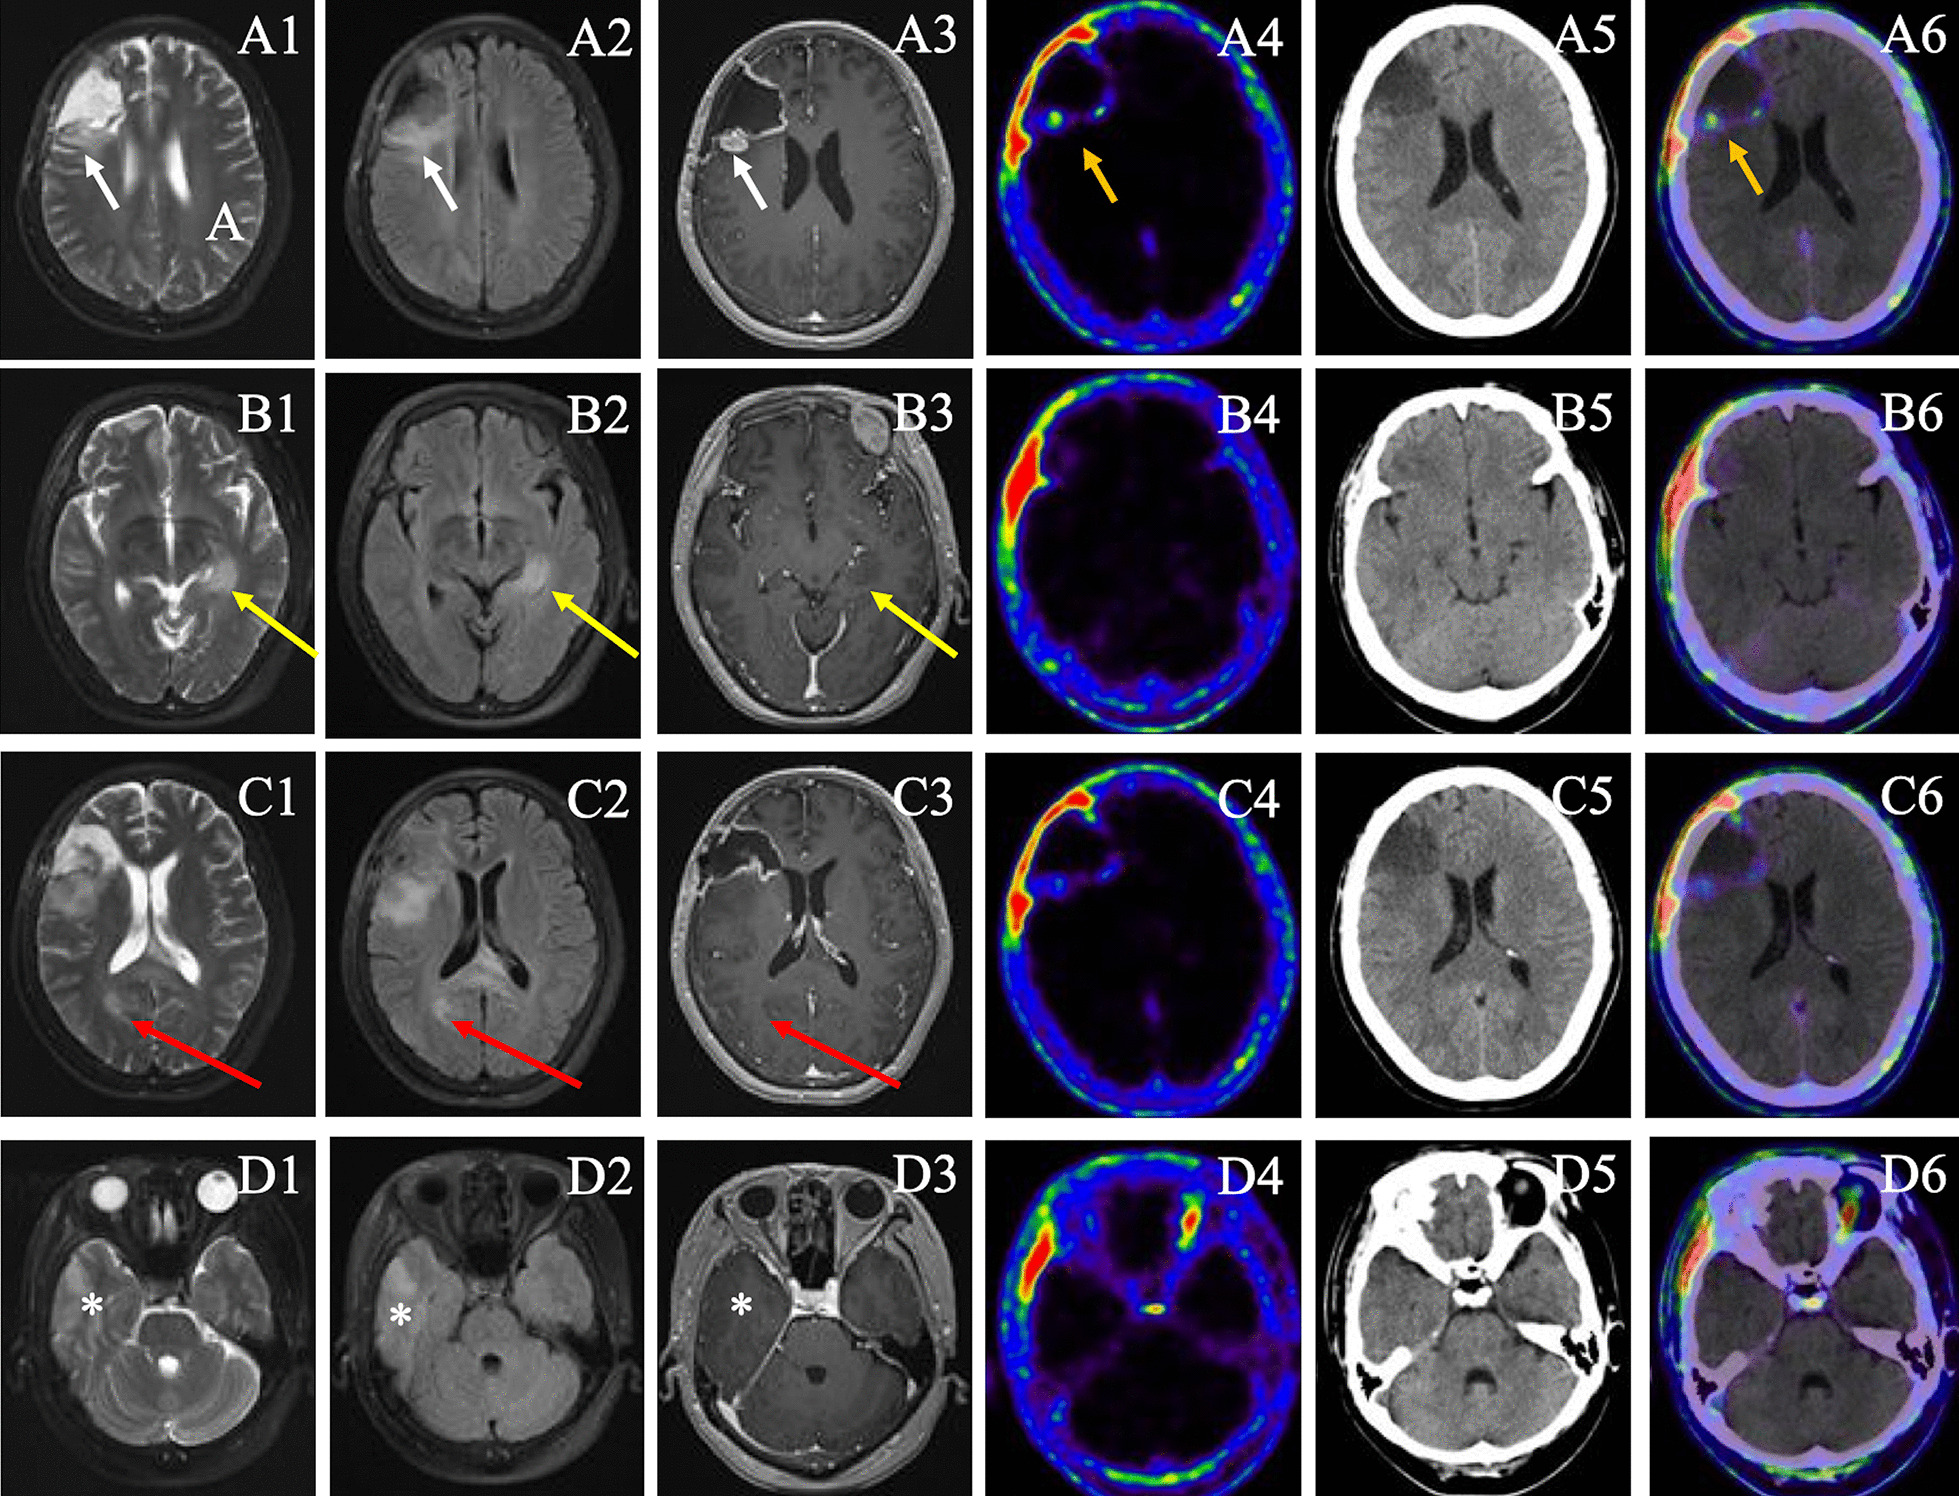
The ^18^ F-FAPI PET/CT and MRI (T2, FLAIR, CE-T1) images of patient 6. A1–D1 were images of T2 sequence, A2–D2 were images of FLAIR, A3–D3 were images of enhanced T1, A4–D4 were images of ^18^ F-FAPI PET, A5–D5 were images of non-enhanced CT, A6–D6 were images of fusion of ^18^ F-FAPI PET and CT. The patient underwent incomplete surgical resection of the glioblastoma in the right frontal lobe. Four lesions were detected on MRI (A1-D1, short arrow, yellow arrow, red arrow, and asterisk). Only one ^18^ F-FAPI-avid lesion was observed on PET/CT (orange arrow, A4-A6).
